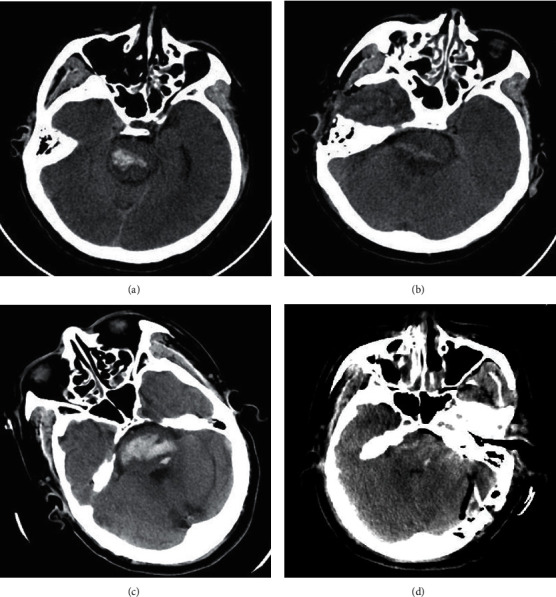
Forty-eight years old, male, who was admitted to the hospital with sudden unconsciousness for 3 hours. Physical examination: The patient was in coma with respiratory failure and tracheal intubation. Bilateral pupils were 1.5 mm, and the light reflex was negative. The patient was quadriplegic and bilateral Babinski's sign positive. Preoperative brain CT showed that the brainstem hematoma was located in the upper part of the pons, which was of unilateral tegmental type (a). The hematoma was cleared by subtemporal approach. Brain CT at 24 hours after operation showed that pons hemorrhage was cleared satisfactorily (b). Fifty-four years old, male, admitted to the hospital with sudden unconsciousness for 1 hour. Physical examination: The patient was in coma with bilateral miosis and negative for light reflex. The patient presented with quadriplegia, hypotonia, and bilateral Babinski's sign positive. Preoperative brain CT showed that the hematoma was located in the middle and lower part of the pons, which was of bilateral tegmental type (c). The hematoma was cleared through the retrosigmoid sinus approach. Brain CT at 24 hours after operation showed that pons hematoma was completely cleared and the fourth ventricle was clear (d).
